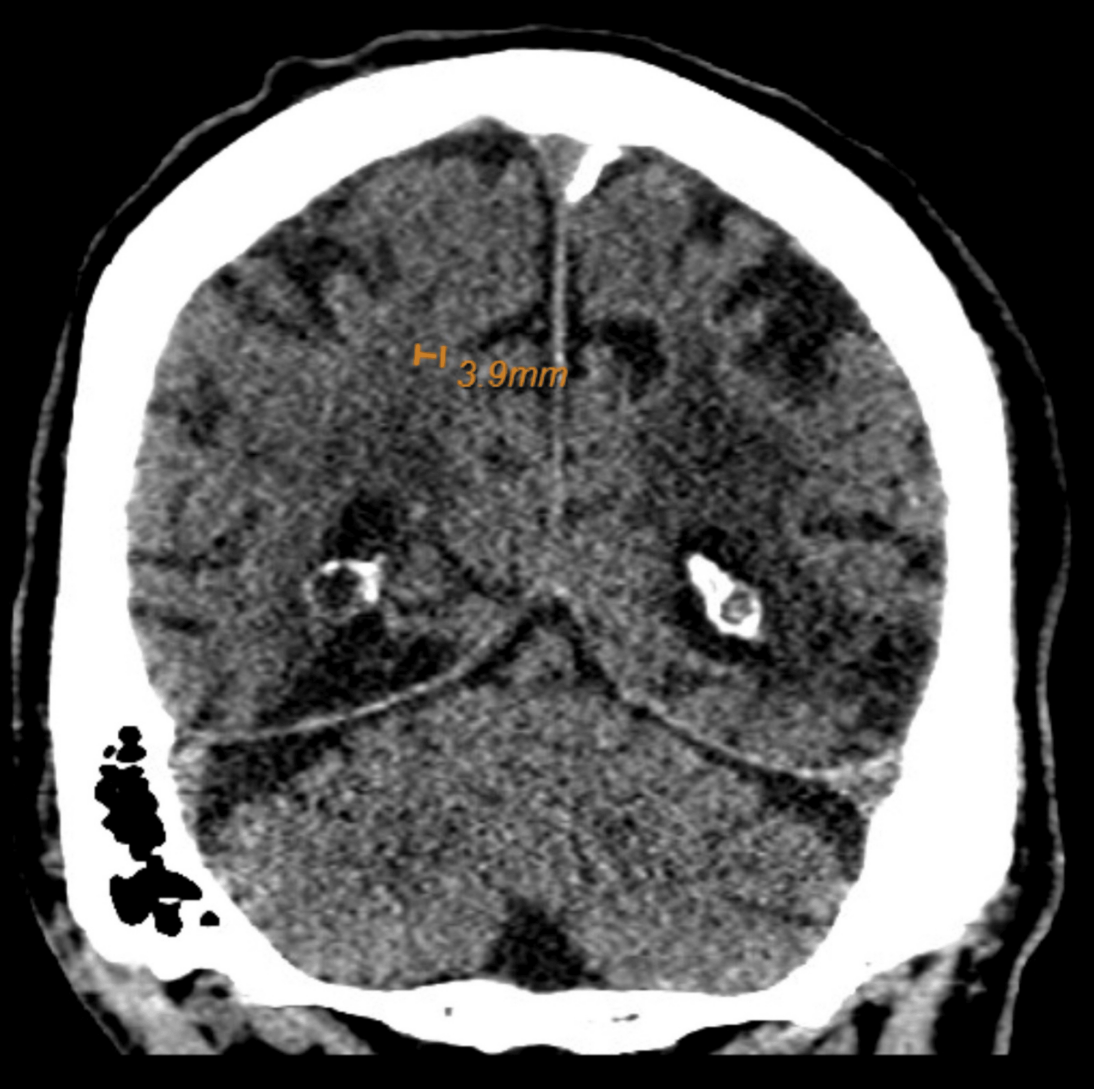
Computed tomography of the brain on day 16 of hospitalization: diminished size of a high right parietal focus of hyperattenuation measuring 4 mm, consistent with the evolution/resolution of parenchymal hemorrhage.
